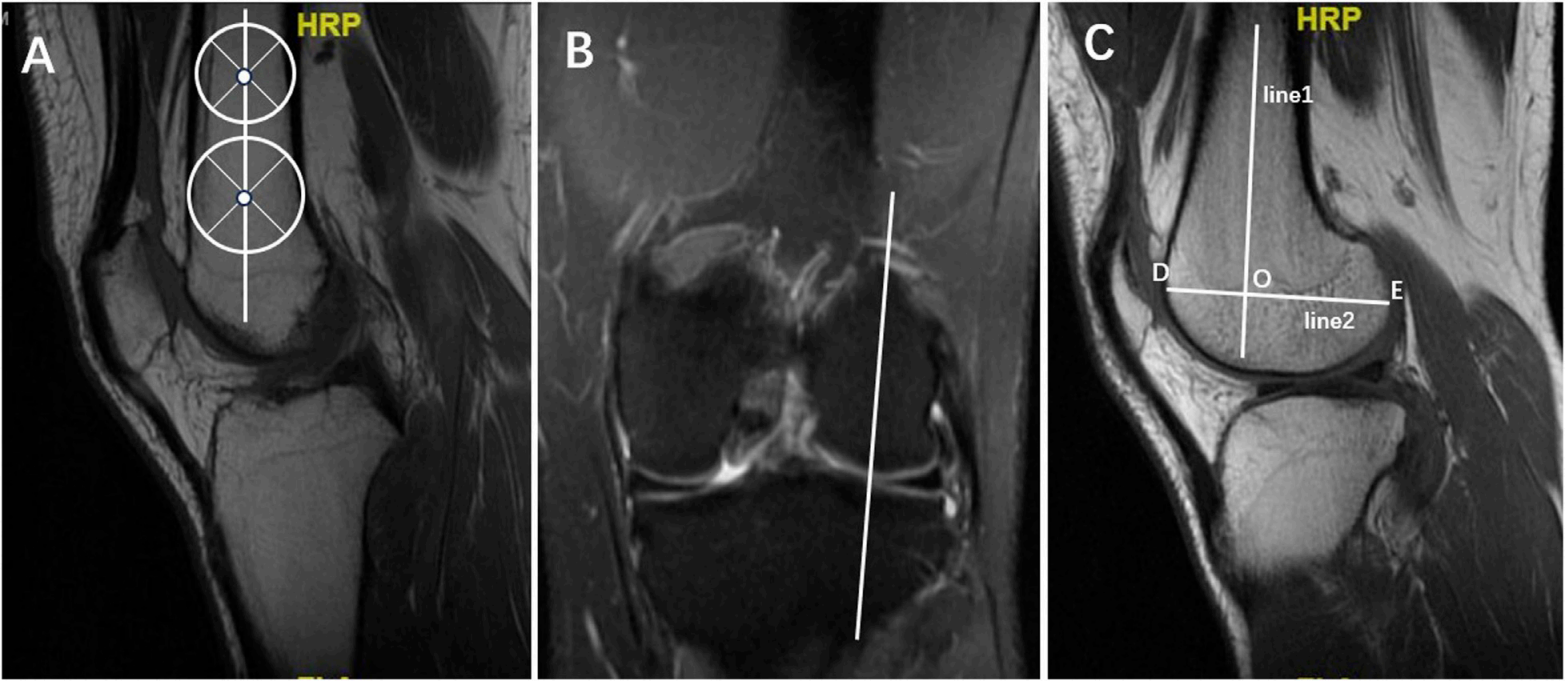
Lateral femoral condyle ratio (LFCI) measurement. (A) In the sagittal T1 centre of the knee MRI, two circles were drawn at the center of the distal femur. The more distant circle should be as close as possible to the trochlea of the femur. A line that passes through the centers of the two circles was thus used to determine the long axis of the distal femur (line1). (B) On T1 coronal sections, the midsagittal plane of the lateral femoral condyle was determined at the level of the popliteal groove. (C) On the corresponding sagittal slide (T1), the long axis of the distal femur (line1) was replicated and another line was drawn between the most anterior point (point D) and the last point of the lateral condyle (point E) to determine the long axis of the lateral condyle of the femur (line2). The distance from the intersection (point O) of these two lines to the last point of the lateral condyle was divided by the total length of the lateral condyle. LFCR = OE/DE.
